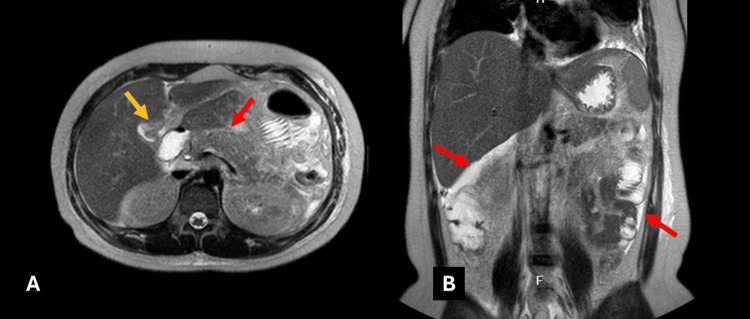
Contrast-enhanced MRI (abdomen): Axial T2-weighted sequence (A) shows mild pancreatic hyperintensity (red arrow) and biliary sludge (yellow arrow). Coronal T2-weighted sequence (B) shows free abdominal fluid (red arrows).
